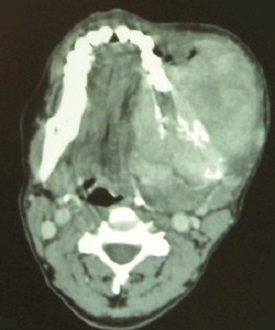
Axial CT scan showing destruction of the bone and soft tissues enhancement that extended buccally and lingually
